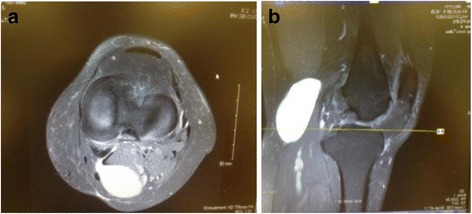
Preoperative MRI image of the left knee popliteal cyst. a Axial view shows that the popliteal cyst is located between the medial gastrocnemius and semimembranosus, and there is communication between popliteal cyst and knee cavity. b Sagittal view shows that the popliteal cyst is located posteriorly to the medial gastrocnemius
